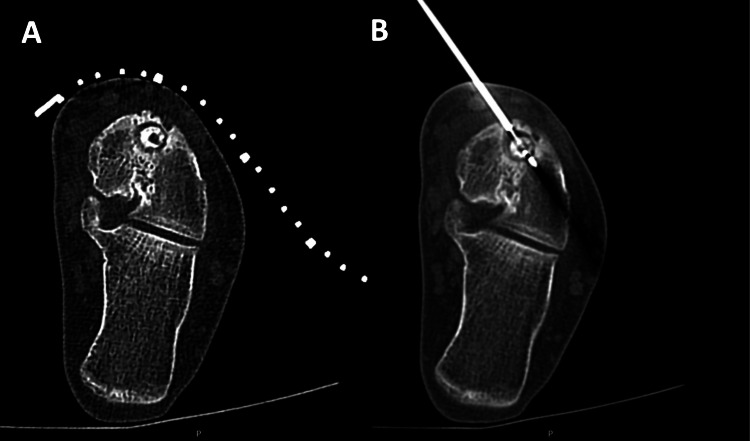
Scans showing CT-guided radiofrequency ablation of an osteoid osteoma in the neck of the talus bone of the left foot A, a pre-ablation scan showing the overlying adhesive localizer grid. B, a peri-ablation scan showing the radiofrequency ablation probe tip positioned inside the nidus. This is a computed tomography (CT) image of one of the patients included in the study.
